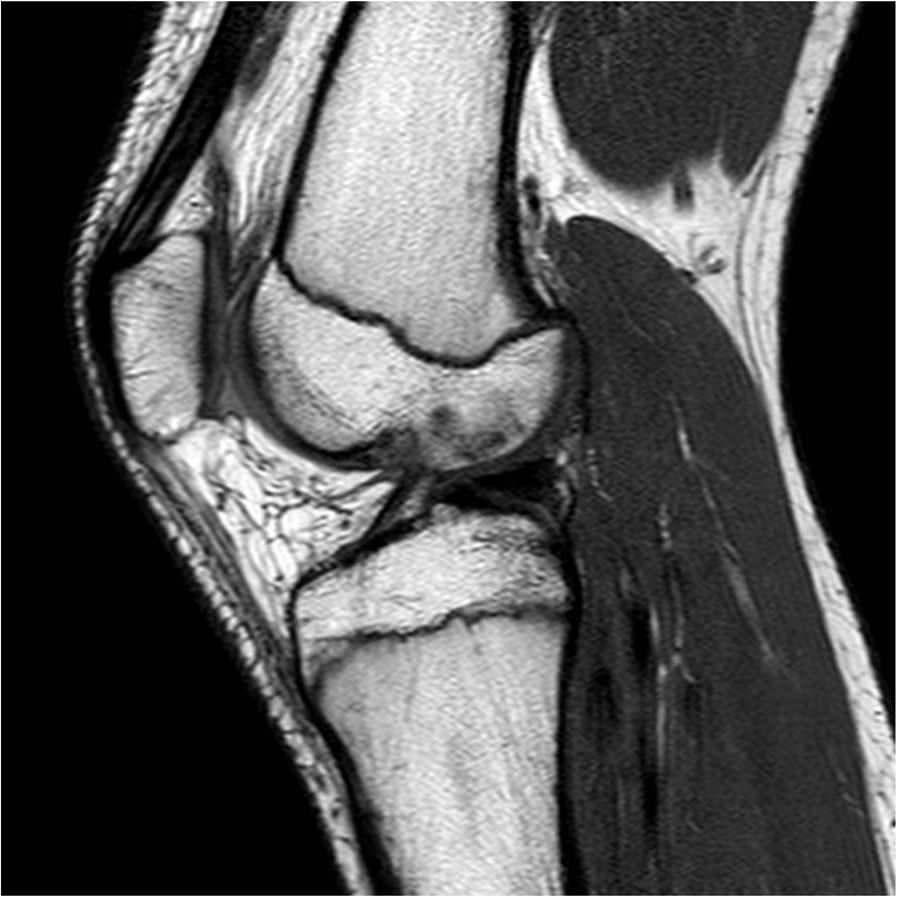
The MRI shows abnormal band originated from the anterior horn of medial meniscus directed to the femoral insertion of the ACL (red arrow)
